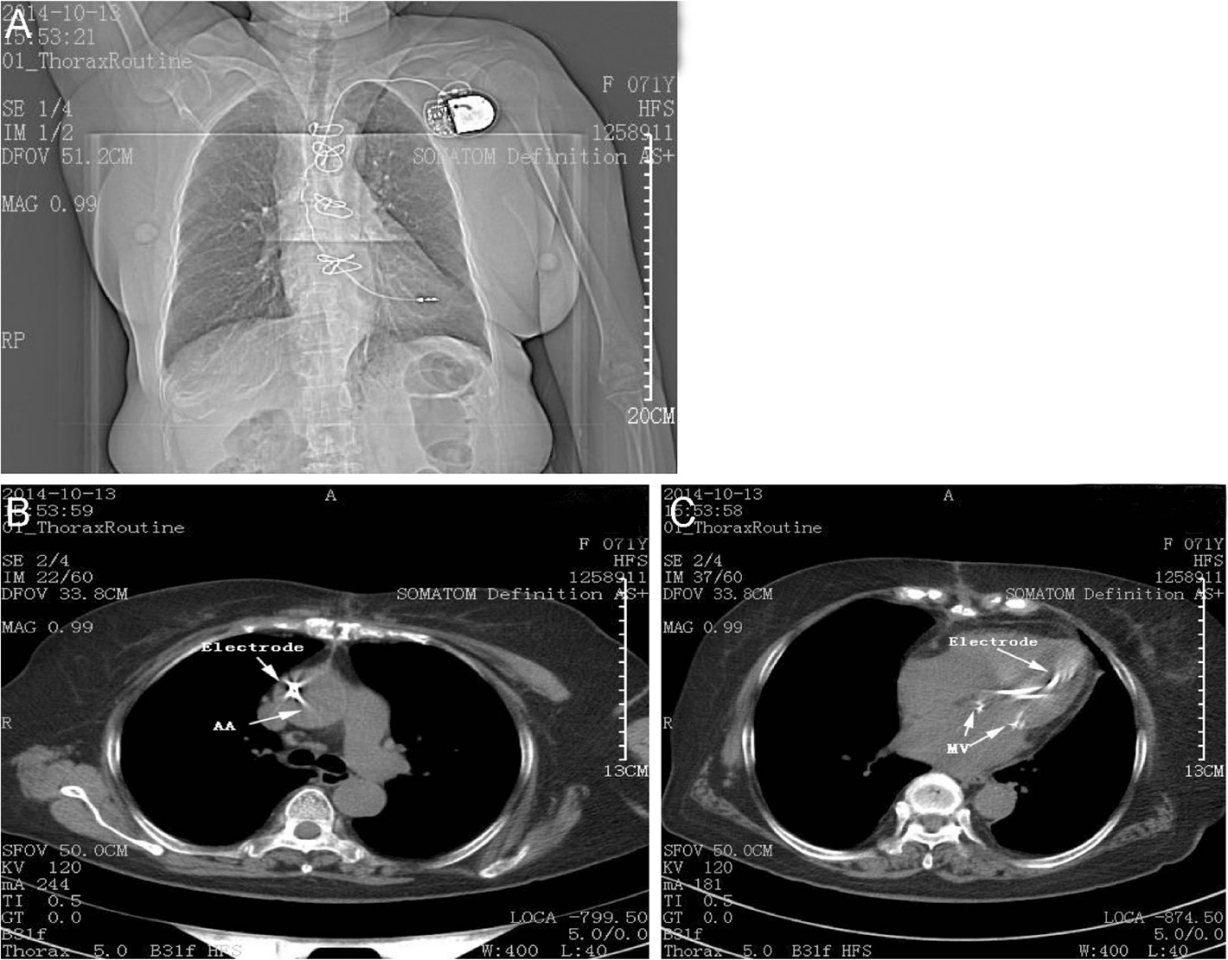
Chest CT after operation. A postoperative state of cardiac pacemaker implantation. B Chest CT scanning indicated pacing electrode in ascending aorta. C Chest CT scanning indicated the pacing leads in left ventricle
